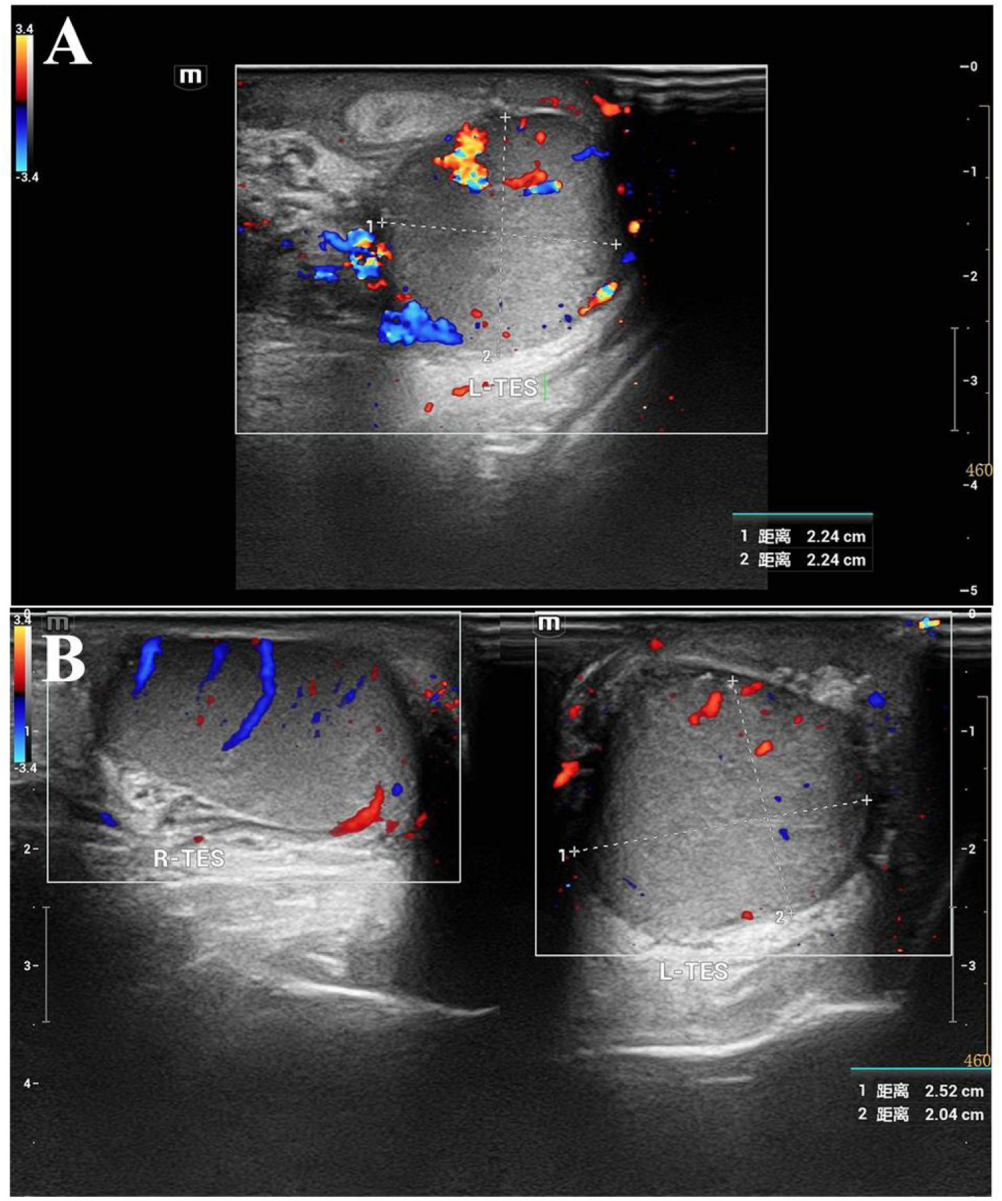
Postoperative follow-up inguinal and scrotal testicular ultrasound findings; (A) left testis, full shape, testis and epididymis have acceptable echo, and intra-testicular blood flow signals are visible; (B) comparative scanning of both testes: the left testis is slightly full, and blood flow signals are visible in both testes.
